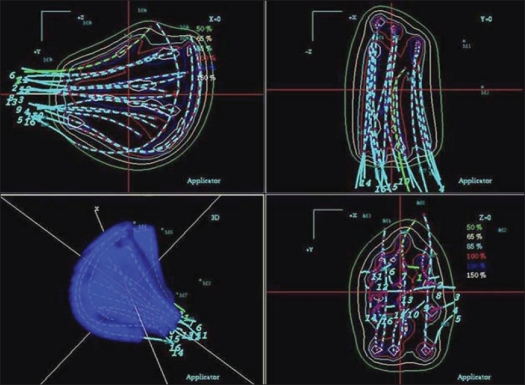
2D and 3D dose distribution resulted from radiograph generated plan
